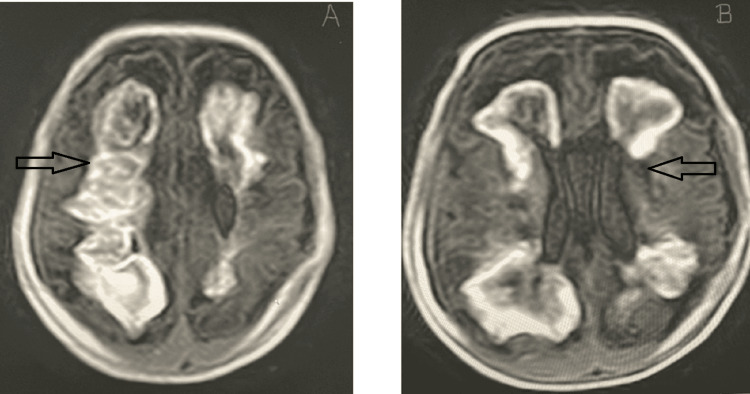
(a) T1-weighted images depicting heterogenous confluent areas of hemorrhages, and (b) hemorrhage involving periventricular area and corona radiata in case 10
